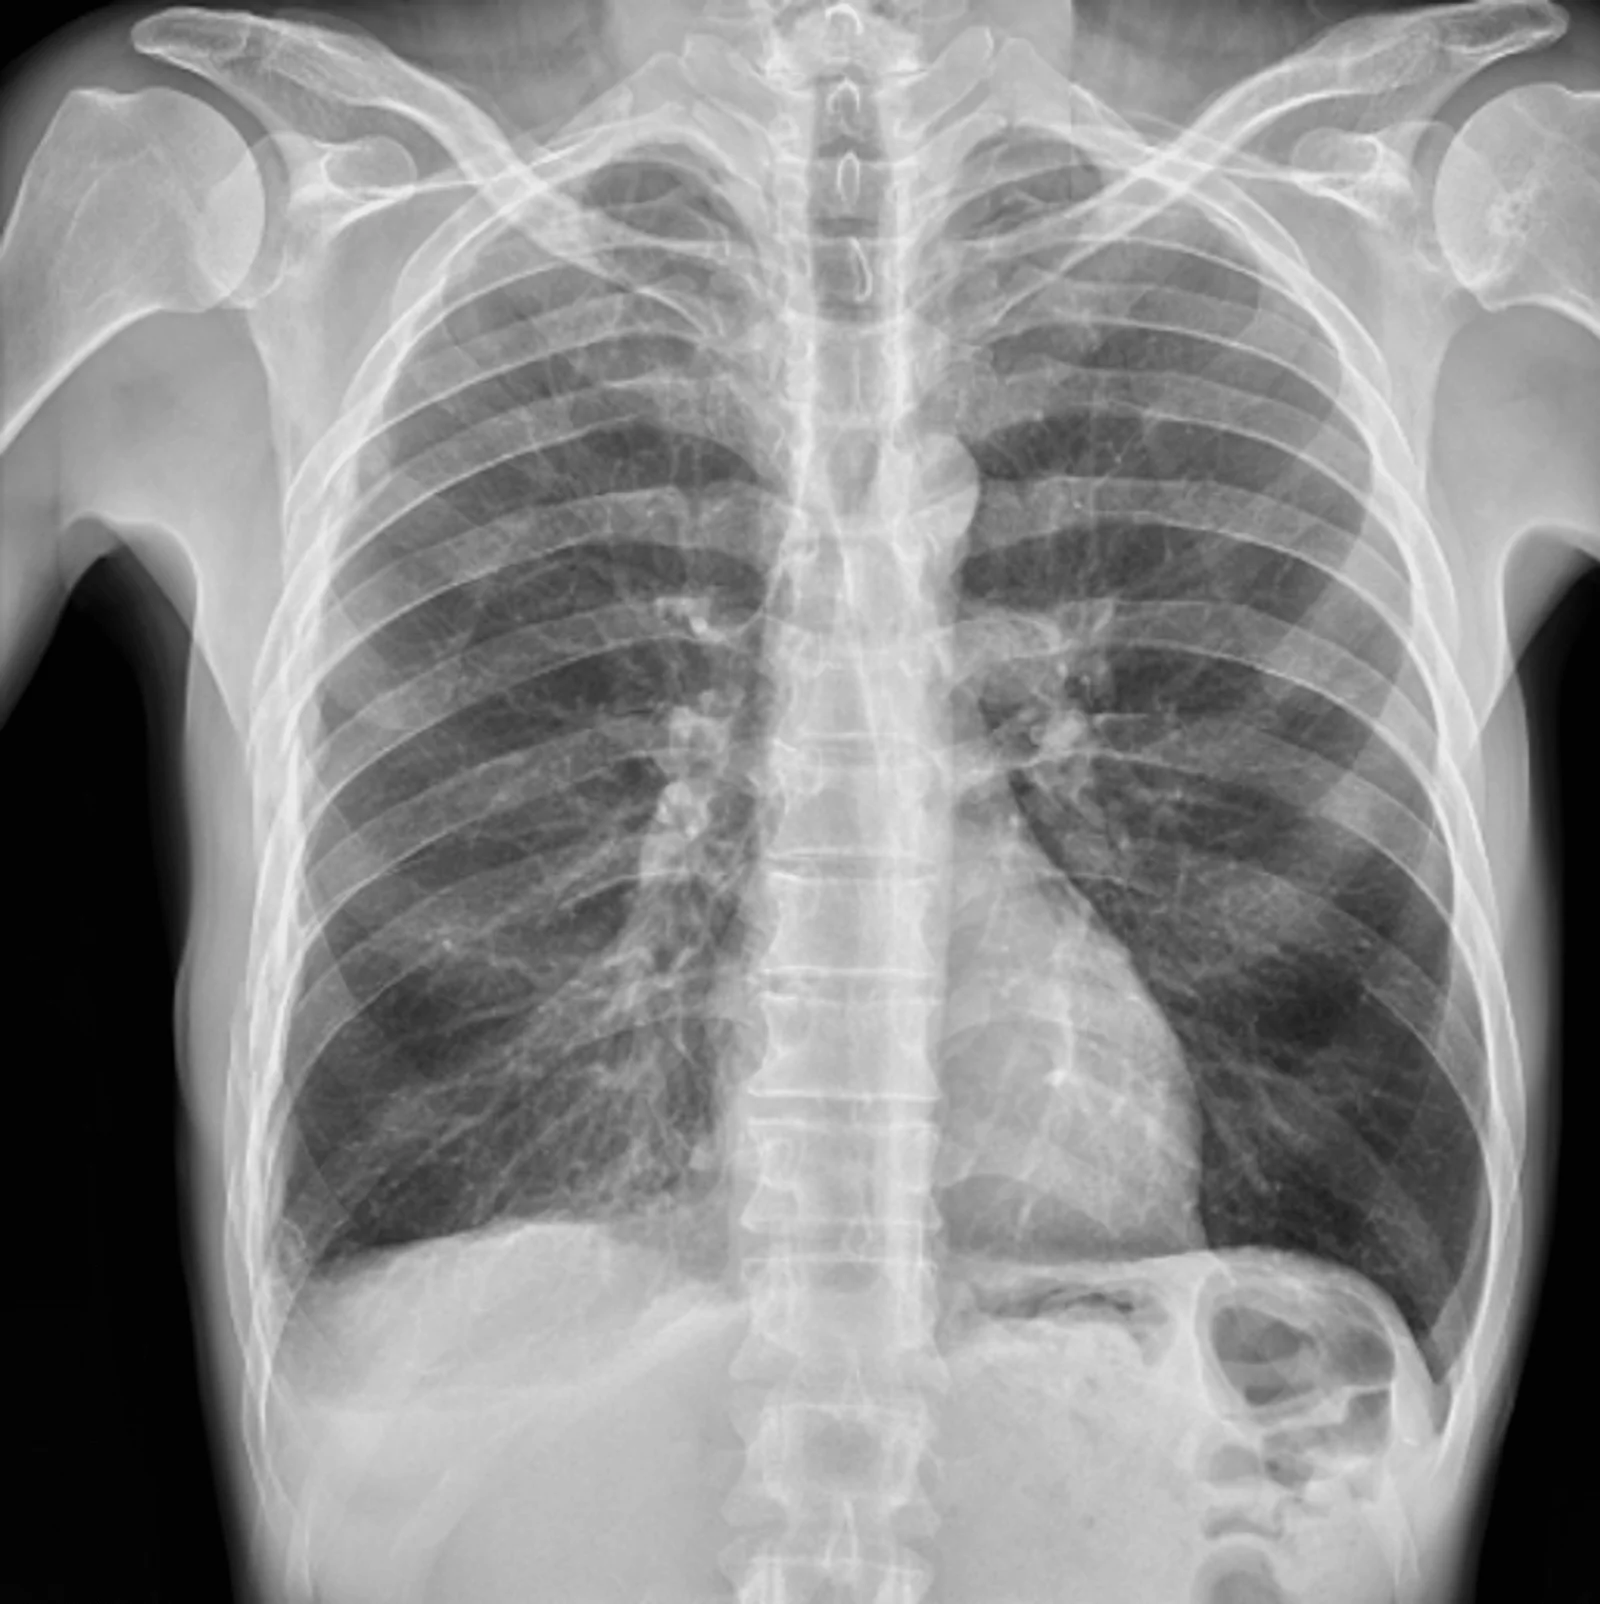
Anteroposterior chest radiograph at two-week post-discharge outpatient follow-up. An upright chest radiograph obtained during the patient's two-week outpatient follow-up visit demonstrates complete resolution of the right-sided hemopneumothorax, with clear lung fields and blunting of the right costophrenic angle.
